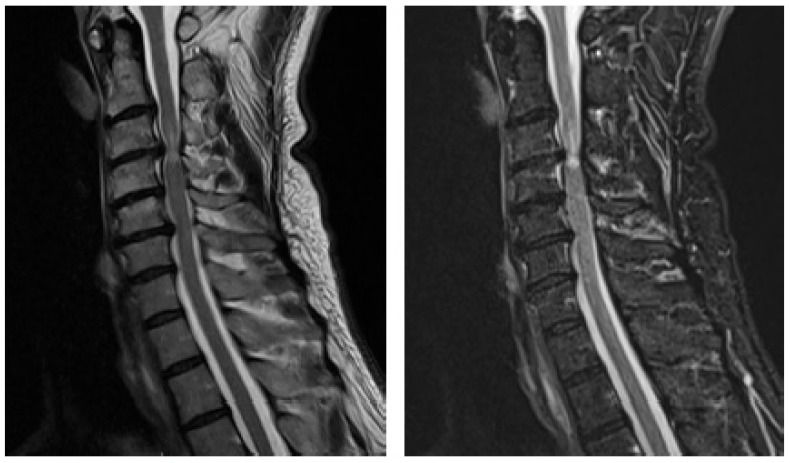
Pre-operative sagittal T2-weighted MRI of the cervical spine demonstrating severe cervical spinal cord compression at C3–4. Sagittal T2-weighted MRI of the cervical spine showing degenerative disc disease at the C3–4 level with significant anterior spinal cord compression and associated intramedullary T2 hyperintensity. The increased cord signal is consistent with myelomalacia and correlates with the patient’s progressive neurological deficits, including gait instability, upper limb clumsiness, and sensory disturbance, supporting the indication for surgical decompression.
